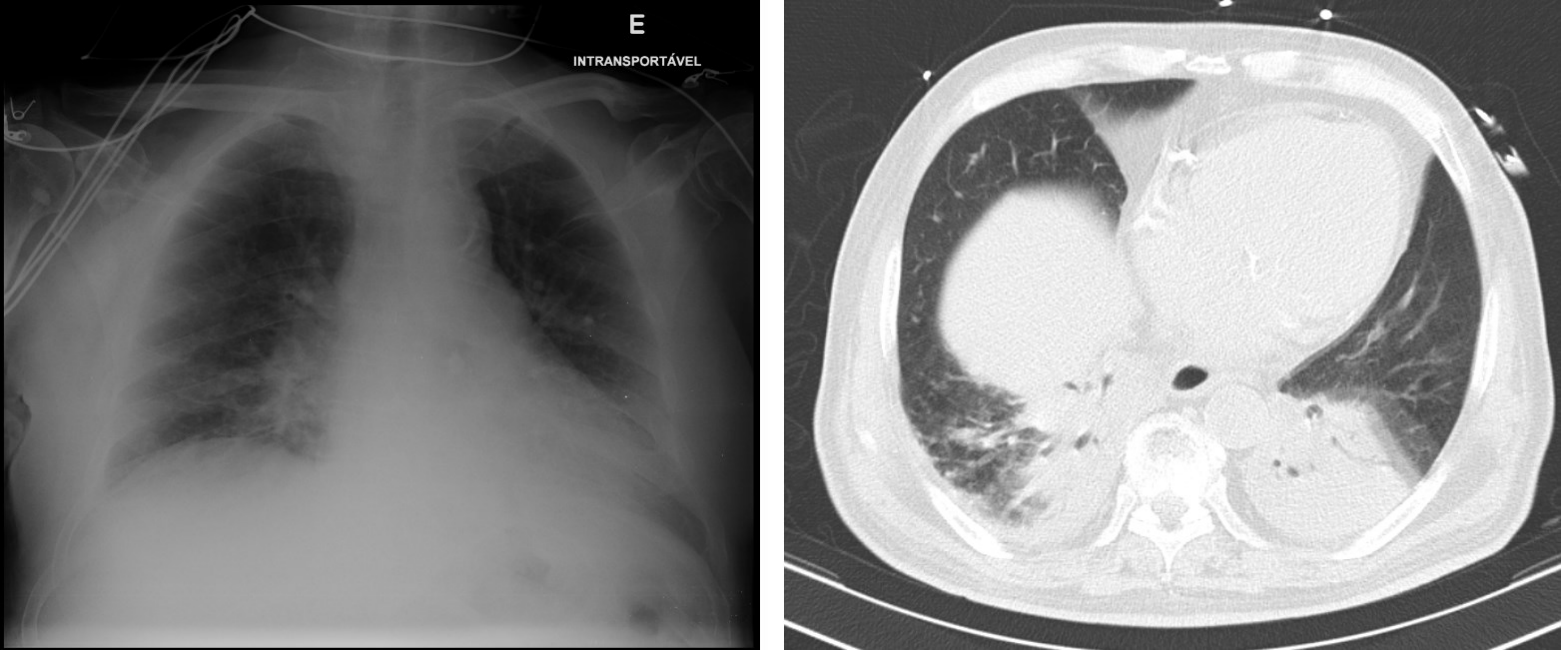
Chest x-ray of the patient (left) and CT scan of same patient (right), performed in the same day, showing right lower lobe consolidation, as well as, left lower lobe consolidation.
